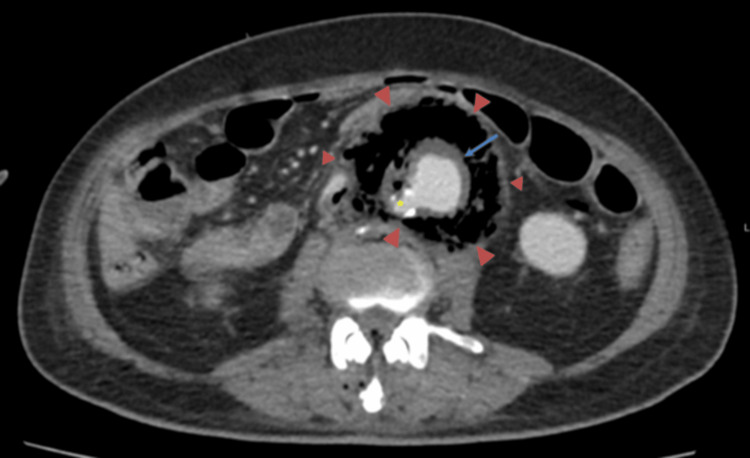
An axial view of the CTA aorta showing a periaortic collection that is filled with air locules (red arrowheads). The abdominal aorta with atherosclerosis (yellow asterisk) with a saccular aneurysmal dilatation (blue arrow) is noted. CTA: Computed tomography angiography
